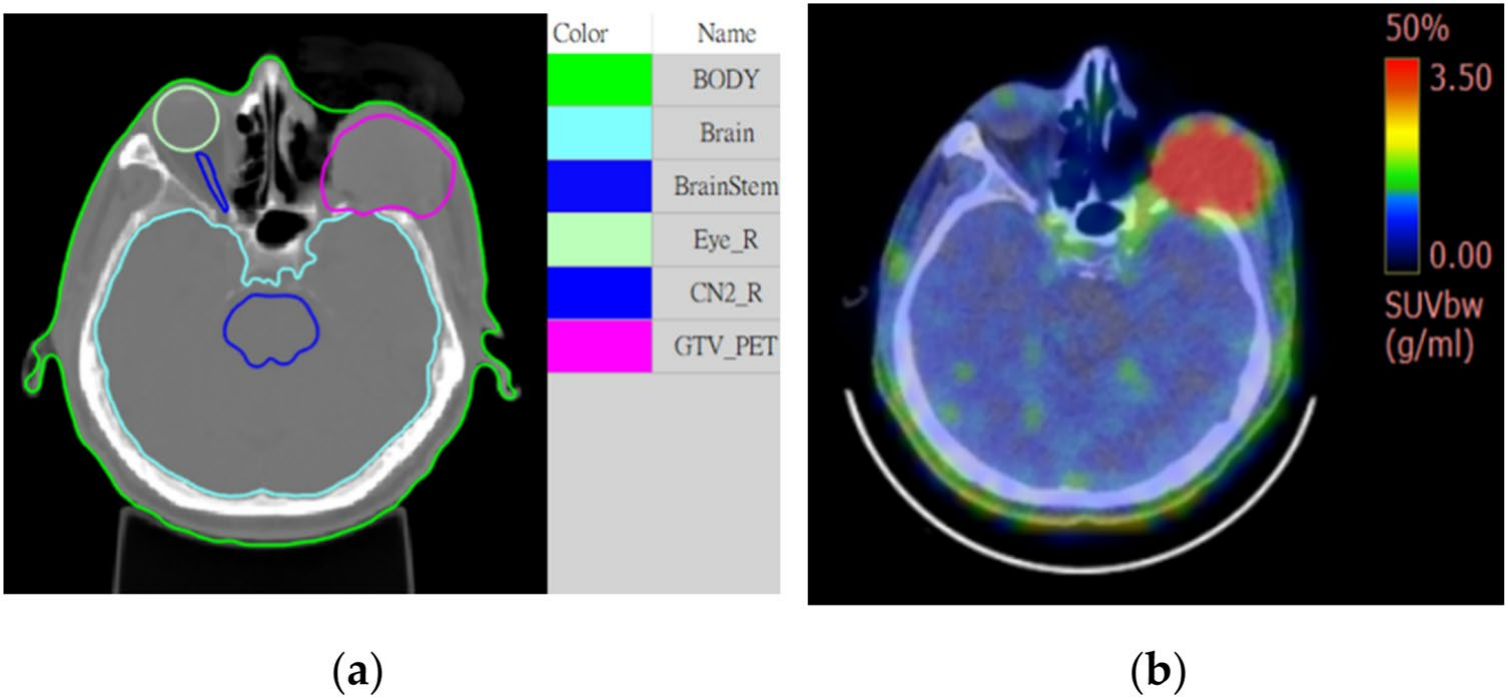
(a) Shows the computer tomography (CT) image with ROI information retrieved from the RTSTRUCT file, where the tag Brain represents the normal brain, and the tag GTV_PET represents the tumor region, which is defined based on the PET image. (b) F-BPA PET/CT fusion image.
